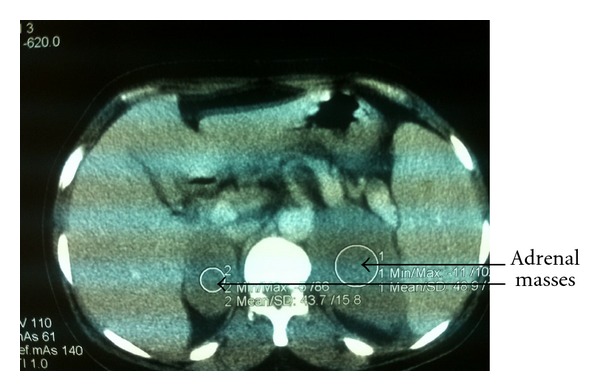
The abdominal CT scan demonstrated frankly bilateral volumes of adrenal masses.
